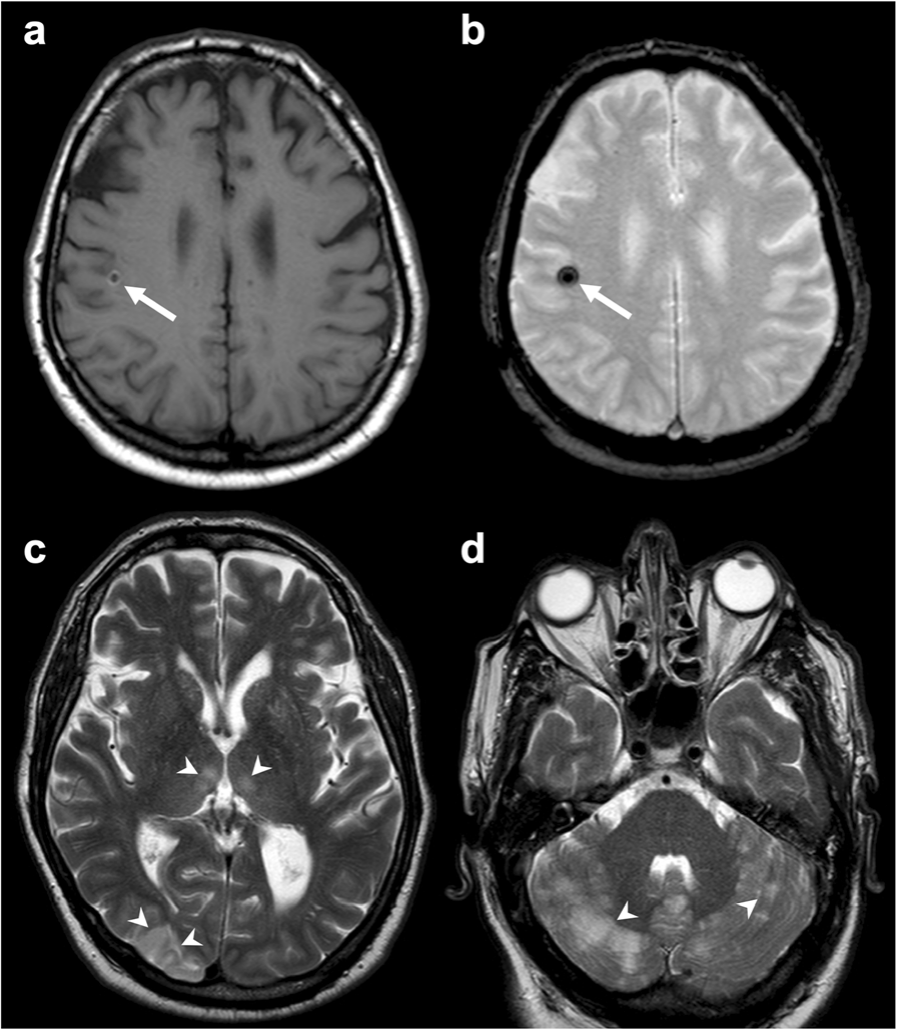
Brain Teflon embolism. Axial T1-weighted (a), susceptibility-weighted (b), and T2-weighted (c, d) images depicting multiple ischemic lesions (arrowheads c, d) due to Teflon emboli (arrow in a and b) subsequent to aortic dissection surgery
